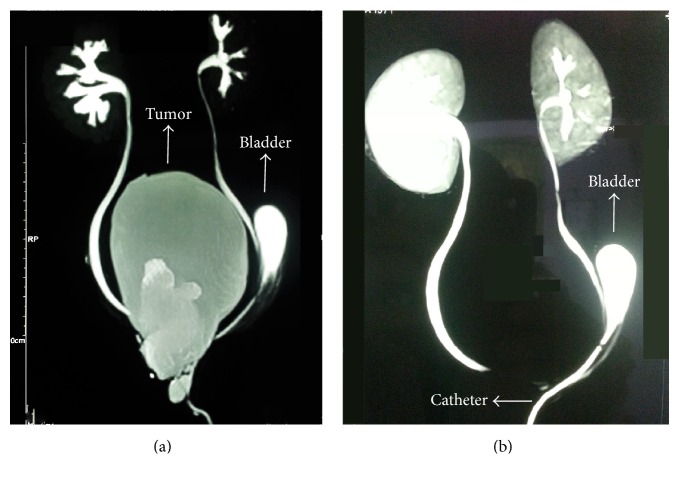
(a) Static magnetic resonance urogram showing the tumor displacing the bladder. (b) Functional magnetic resonance imaging showing ureters, displaced bladder, and hydronephrosis in both kidneys.
